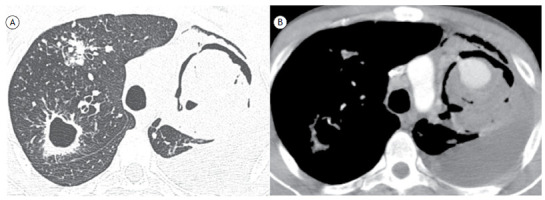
In A, CT scan of the chest with lung window settings at the level of the upper lobes, showing nodules and a thick-walled cavity in the right upper lobe. On the left, note a large cavitary lesion and an intracavitary mass, with air interposed between the mass and the cavity wall (the air crescent sign). In B, CT scan of the chest with mediastinal window settings after administration of intravenous contrast, showing intense enhancement of the intracavitary mass, consistent with a diagnosis of aneurysm formation (Rasmussen’s aneurysm).
